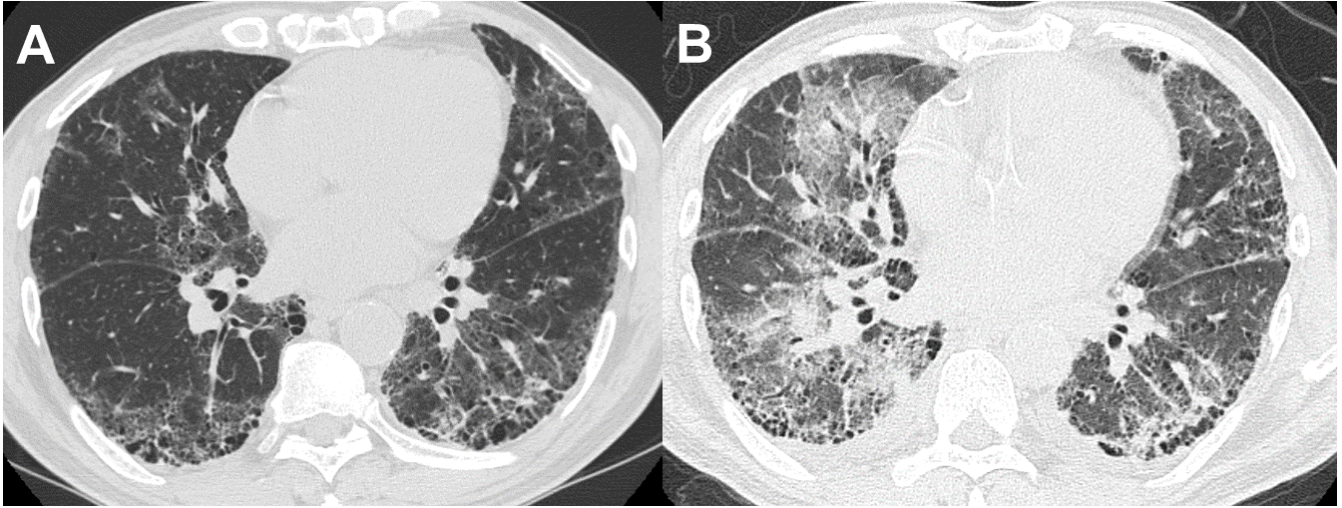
A patient in their 60s with interstitial pneumonia with connective tissue disease. (A) HRCT before the BAL procedure showed small cysts and reticular shadows. (B) HRCT at 20 days after the BAL procedure showed extensive ground-glass opacities in other areas.
